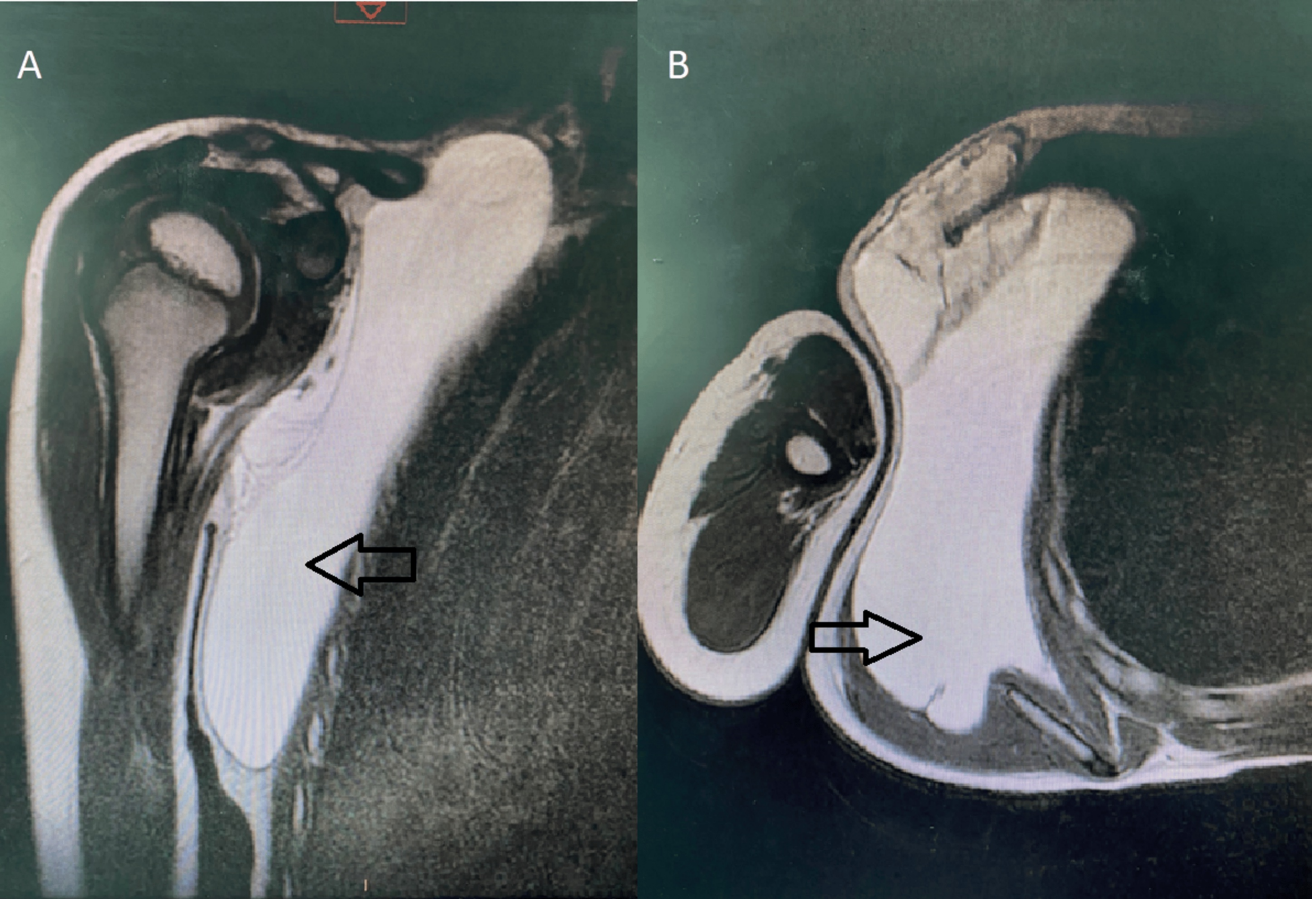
(A) Coronal magnetic resonance imaging showing a superficial swelling (arrow) with a fluid signal content. (B) Transverse magnetic resonance imaging slice revealing cystic swelling (arrow) of the right chest wall with regular seams on hypersignal T2.
